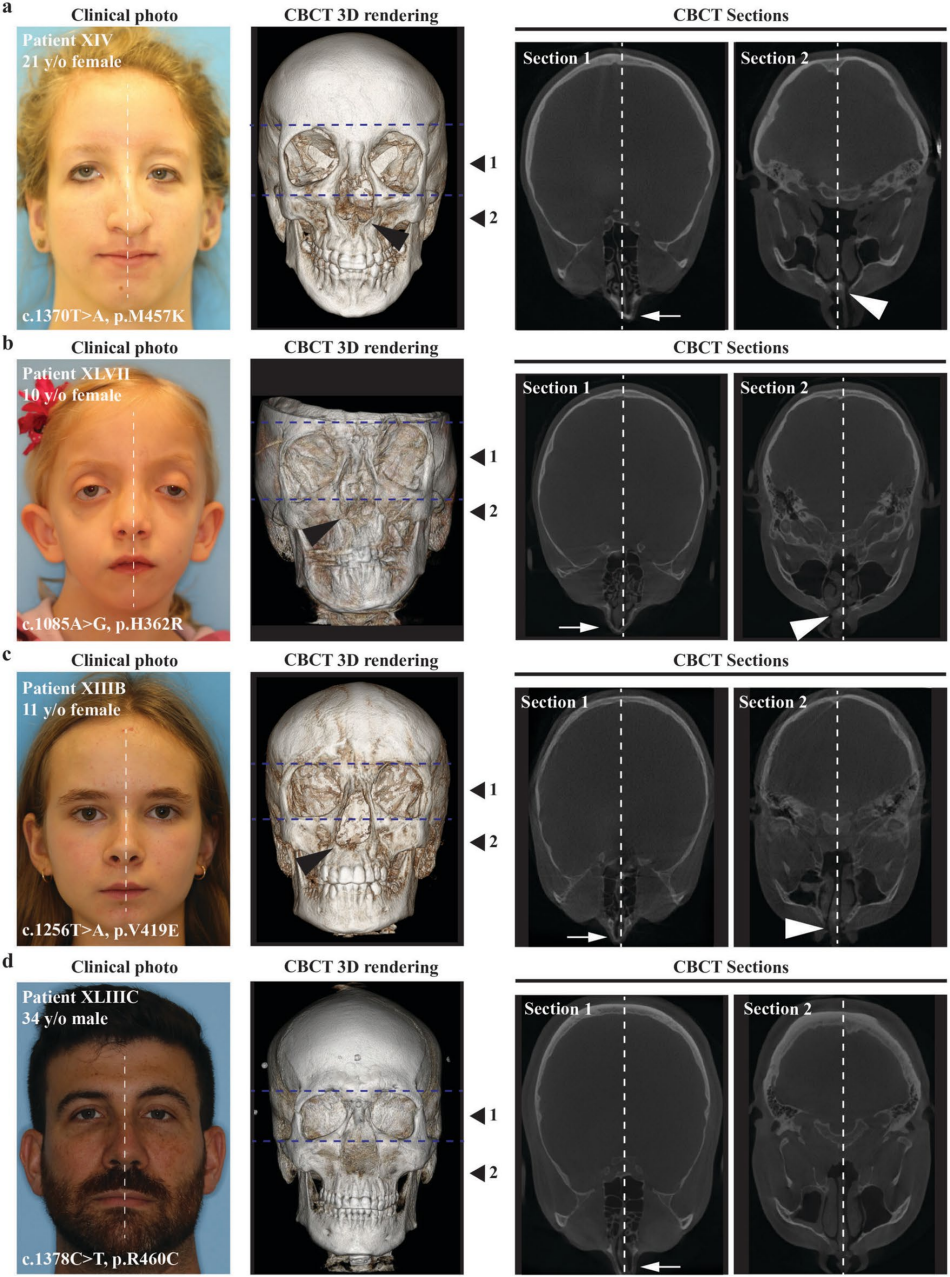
Severe deviation of the nasal tip and abnormal eye shape in females with LDS2. (a) Clinical photo, CBCT 3D reconstruction and section images from a 21-year-old female with *TGFRB2*:c.1370 T > A mutation resulting in a methionine-to-lysine substitution at amino acid 457 (p:M457K) in the protein kinase domain of the receptor. Note the severe deviation of the nasal tip (white arrow) and the skeletal deviation of the nasal bone and the base of the nasal cavity (black and white arrowheads). Abnormal eye shape is due to misalignment of the orbits (blue dotted lines). The positions of Sect. 1 and Sect. 2 are indicated on the right side of the CBCT 3D rendering. (b) Same as A for a 10-year-old female with *TGFBR2*:c.1085A > G mutation resulting in a histidine-to-arginine substitution at amino acid 362 (p.H362R) in the protein kinase domain of the receptor. (c) Same as A for an 11-year-old female with *TGFBR2*:c.1256 T > A mutation resulting in a valine-to-glutamate substitution at amino acid 419 (p.V419E) in the protein kinase domain of the receptor. Contrary to A and B, this patient exhibits milder deviation of the nasal tip and no eye shape abnormalities. (d) Same as A for a 34-year-old male with *TGFBR2*:c.1378C > T mutation resulting in an arginine-to-cysteine substitution at amino acid 460 (p.R460C) in the protein kinase domain of the receptor. This male patient exhibits normal eye shape and mild deviation of the nasal tip when compared to A and B. Skeletal assessment indicates mild deviation of the nasal bone with no deviation at the base of the nasal cavity.
